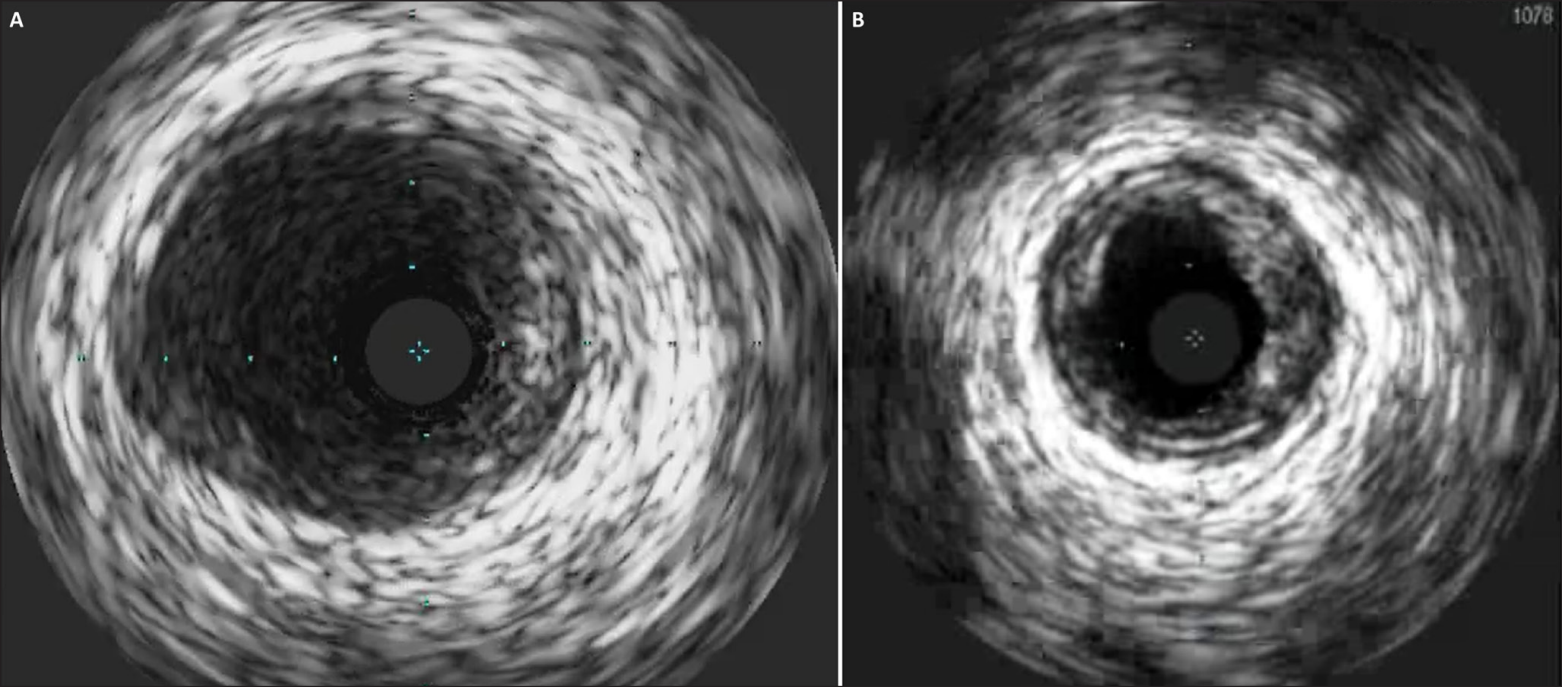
IVUS of the (A) left main coronary artery showing absence of dissection and (B) LCx artery showing absence of dissection with mild to moderate atherosclerotic disease. IVUS: intravascular ultrasound; LCx: left circumflex.
